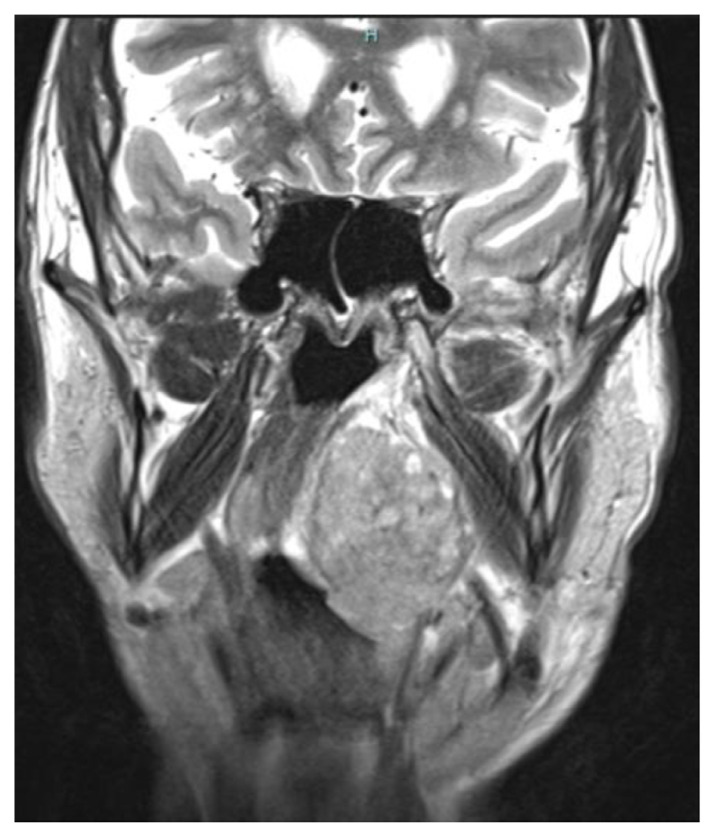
MRI coronal image before chemotherapy.
